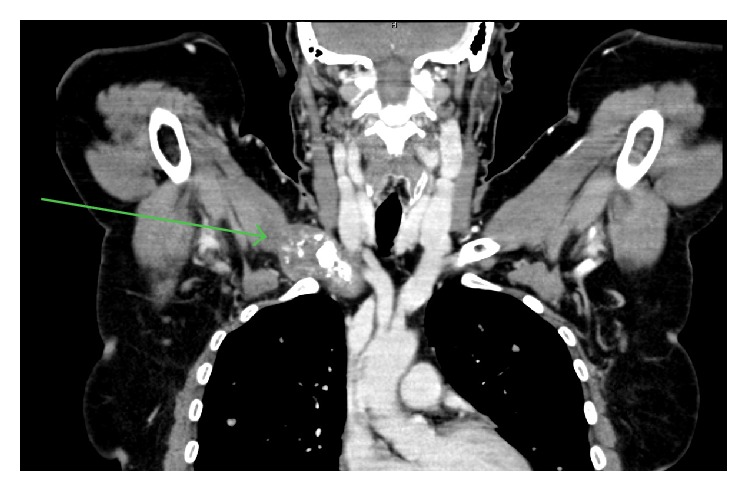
CT scan of thorax (multiplanar reconstruction). The green arrow points to osteolytic metastasis of right clavicle.
